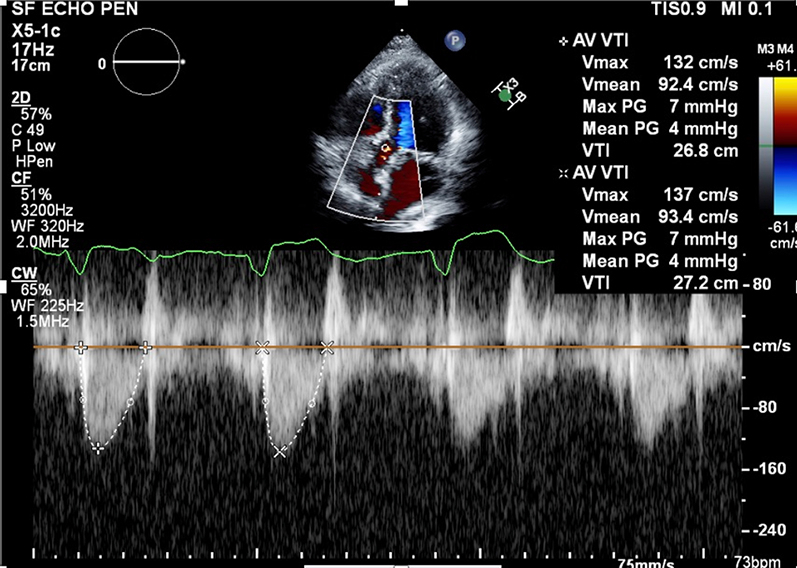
Postprocedural Transthoracic Echocardiogram Assessment CW Doppler across the aortic prosthesis shows normal transvalvular gradients (peak gradient 7 mm Hg, mean gradient 4 mm Hg, V_max_ 137 cm/s). CW = continuous wave Doppler.
